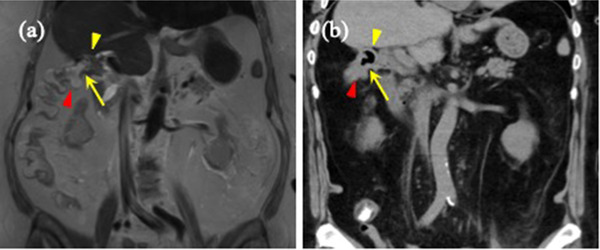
Cholecystocolonic fistula. (a) Magnetic resonance imaging shows the cholecystcolonic fistula (yellow arrow) between the gallbladder (yellow arrowhead) and transverse colon (red arrowhead). (b) Computed tomography showing air in the gallbladder (yellow arrowhead). The positions of the cholecystcolonic fistula (yellow arrow) and transverse colon (red arrowhead) were consistent with magnetic resonance imaging
